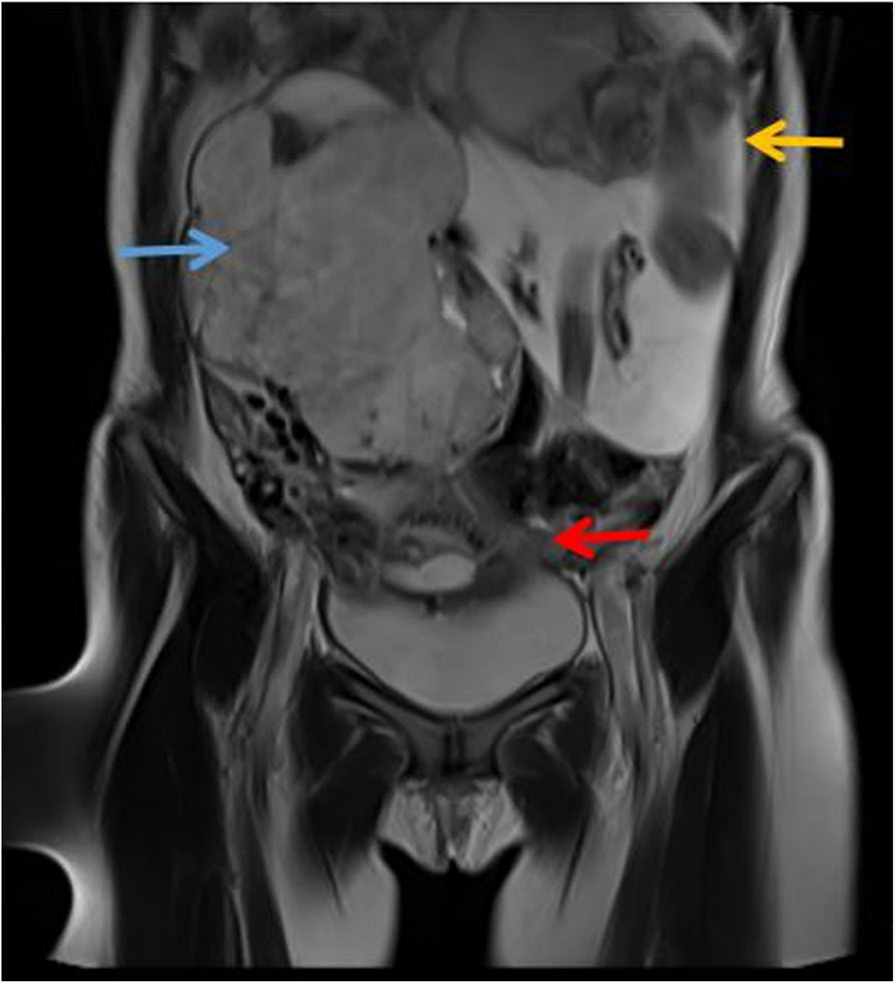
MRI showed a live fetus (yellow arrow) in the abdominal cavity, with the placenta (blue arrow) on the right side of the abdominal cavity rather than in the endometrium (red arrow)
